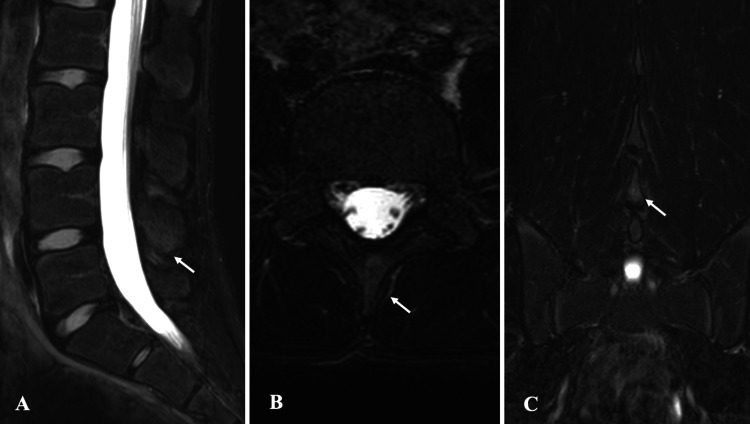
MRI findings in Case 1 three months after treatment. STIR MRI shows complete resolution of high signal intensity at the L4 spinous process: (A) sagittal view, (B) axial view, and (C) coronal view. MRI, magnetic resonance imaging; STIR, short-tau inversion recovery
